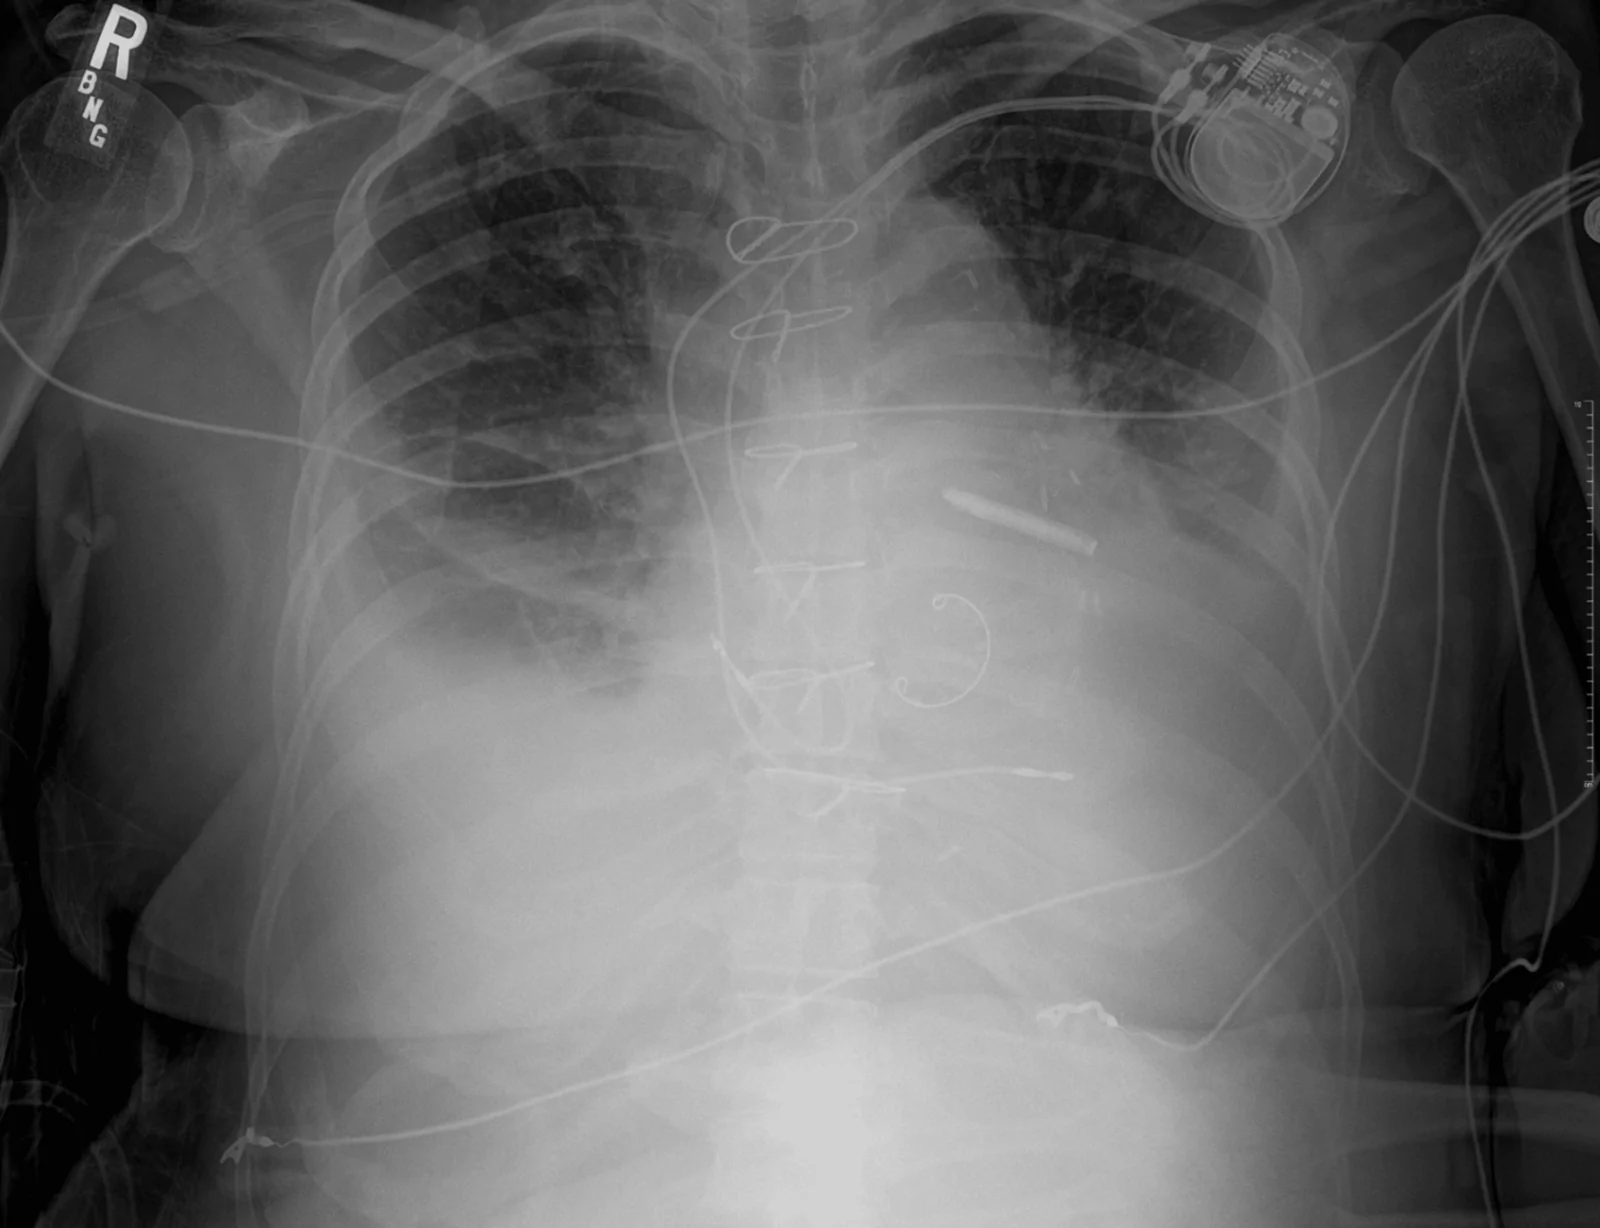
Postoperative chest radiograph on postoperative day 11 demonstrating moderate bilateral pleural effusions with baseline interstitial opacities and retrocardiac consolidation prior to thoracentesis.
